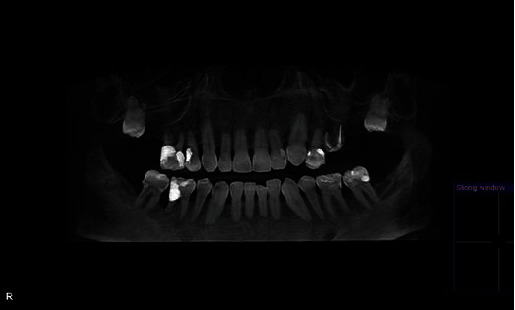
Panoramic radiograph (circa 2016) taken before extractions.
